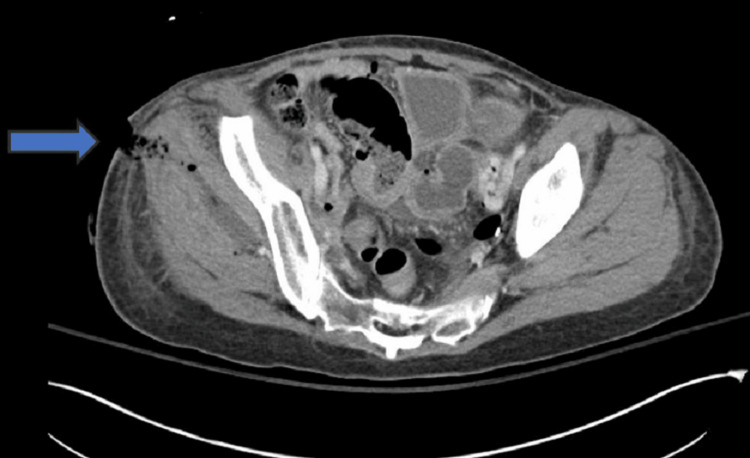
Axial CT image of the pelvis demonstrating progression of the pelvic fluid collection with clear evidence of a fistulous connection between the bowel and the surgical wound. The blue arrows indicate the fistula tract CT: computed tomography
